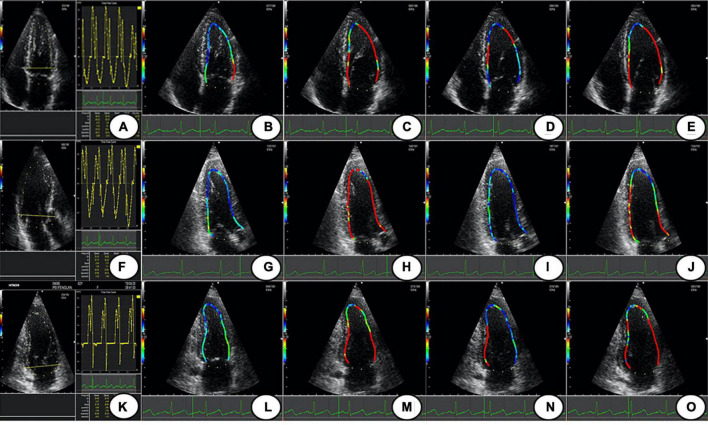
The LV WSS of Apical 4 C, 3C, and 2C; (A,F,K) are the time-flow curves of Apical 4C, 3C, and 2C. (B–E) The LV WSS of Apical 4C in P1–P4; (G–J) the LV WSS of Apical 3C in P1–P4; (L–O) the LV WSS of Apical 2C in P1–P4. Apical 4C, apical 4-chamber; Apical 3C, apical 3-chamber; Apical 2C, apical 2-chamber; P1, isovolumic diastolic period; P2, rapid filling period; P3, slow filling period; P4, atrial contraction period; LV WSS, left ventricular wall shear stress.
